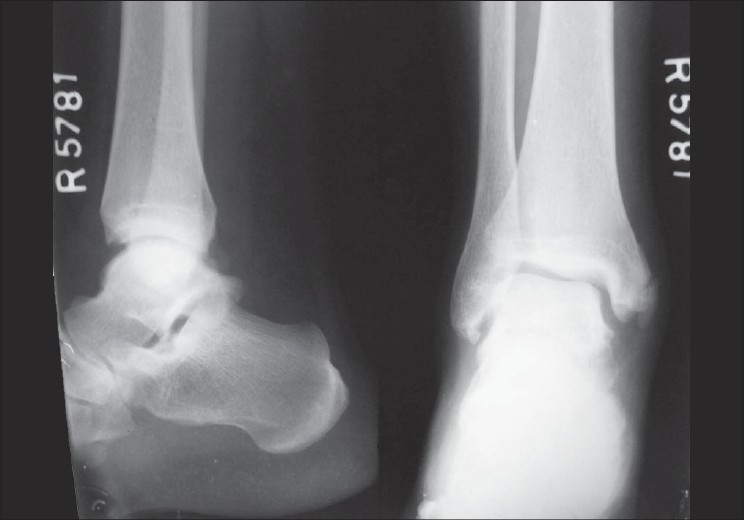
Follow-up X-ray of the ankle revealing osteophytes, calcification of the collateral ligaments beneath the malleoli with mild subluxation of the ankle joint
